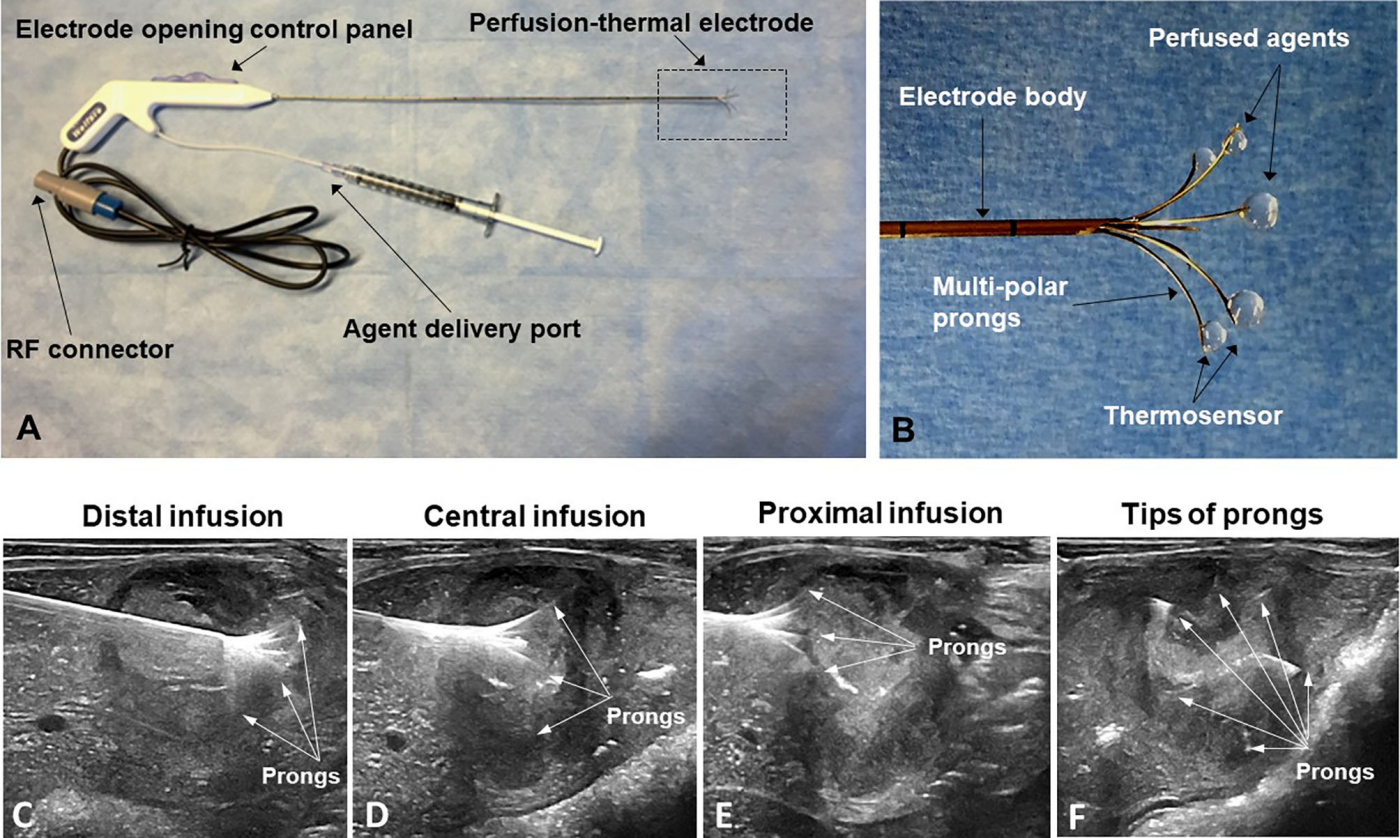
(A, B) The multimodal perfusion thermal electrode with multipolar agent infusion prongs. Micro-thermosensors are equipped at the tips of the prongs for real-time measurement of peritumoral RFH at approximately 42°C, while the central-tumoral RFA tempreature from the electrode body was pre-set at approximately 80°C. (C–F) The stepwise peri-tumor delivery of doxorubicin under real-time ultrasound guidance. The perfusion-thermal electrode is advanced, sequentially into the portion of the tumor most distal from the entry site. At regular intervals during electrode advancement through the tumor, the electrode prongs are opened to the desired size array, with the objective of completely enveloping the tumor peripheral zone for sufficient delivery of the chemotherapeutic agent. During delivery of the chemotherapeutic agent, RFA-mediated RFH at the tumor margin is generated with the same device to further enhance chemo-destruction of tissues at the tumor margins. (A–C) Longitudinal sonographic images; (D) Transverse image.
